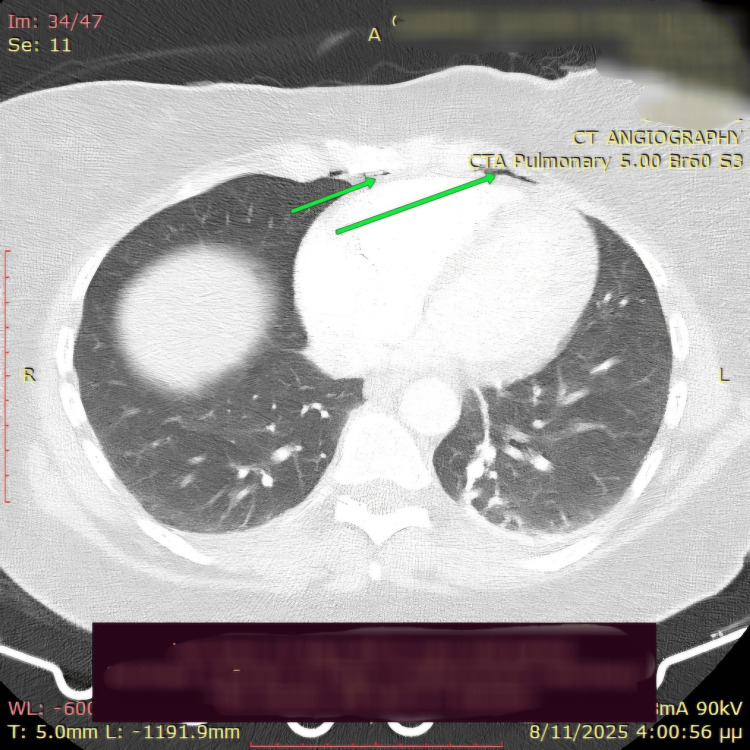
Computed tomography axial image demonstrating pneumomediastinum, with the presence of free air in the mediastinal space (arrows).
